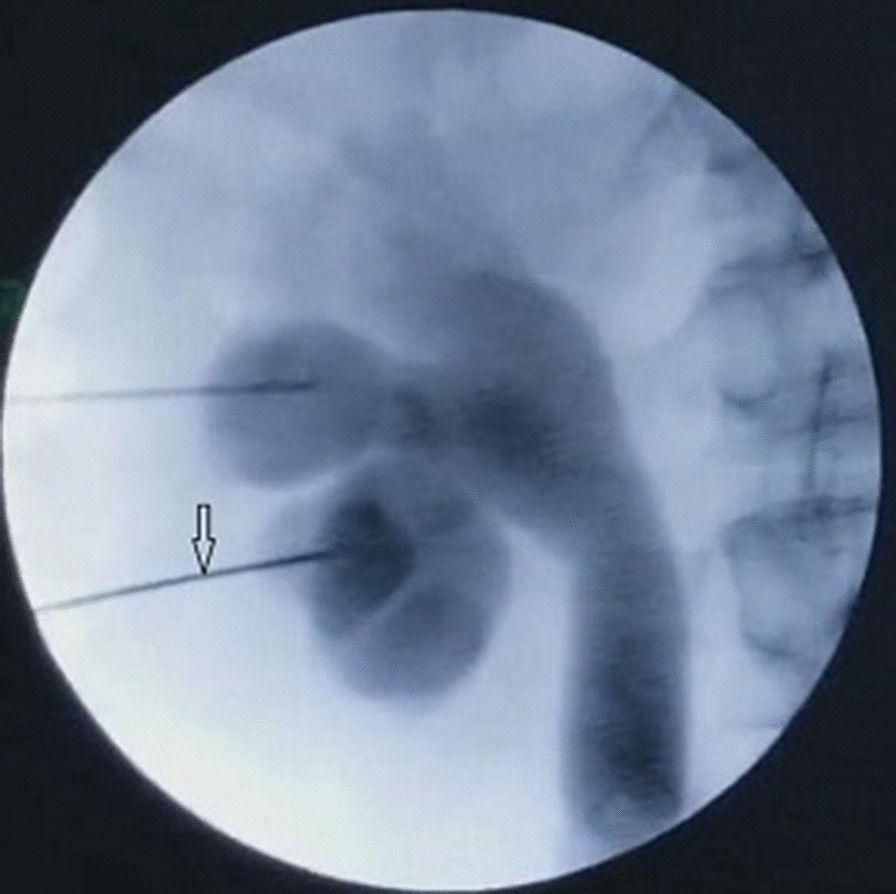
The radiograph of the kidneys, ureters and bladder revealing the collecting system, without visualization of the renal vein by injection of contrast agent through the latter puncture needle (arrow)
